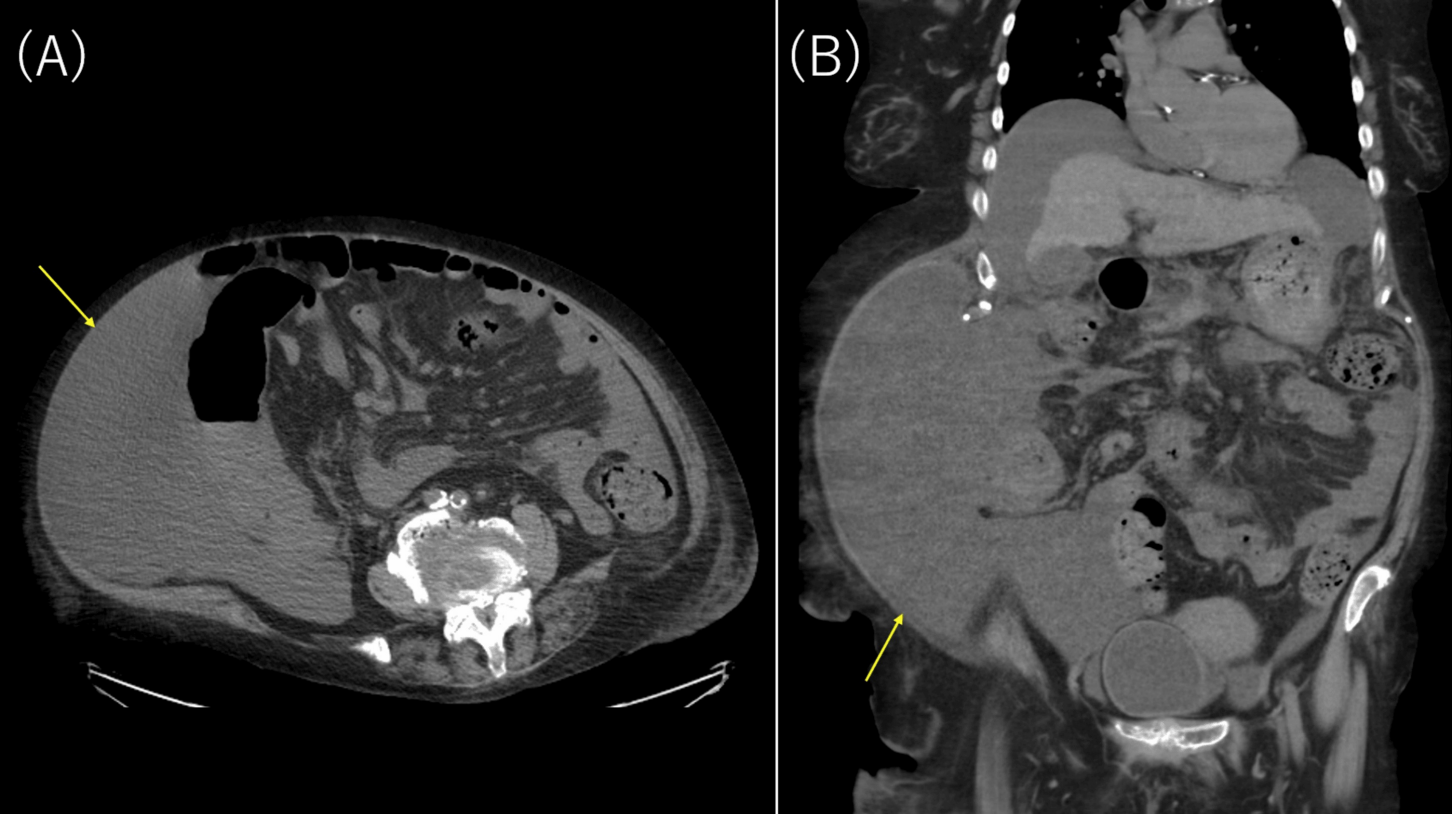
Abdominal CT showing marked ascites (arrows) (A) Axial section, (B) coronal section
